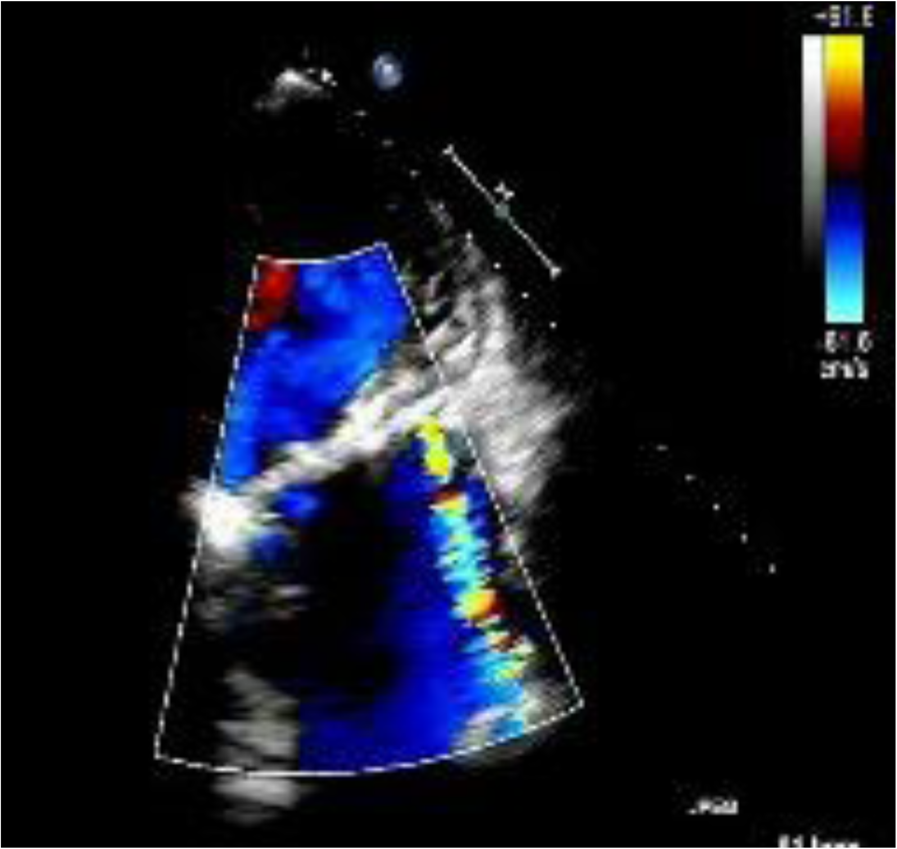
Preoperative echocardiographic findings of a long axis view. Mitral valve regurgitation is apparent around the minor orifice of the implanted valve. The left atrium is enlarged
